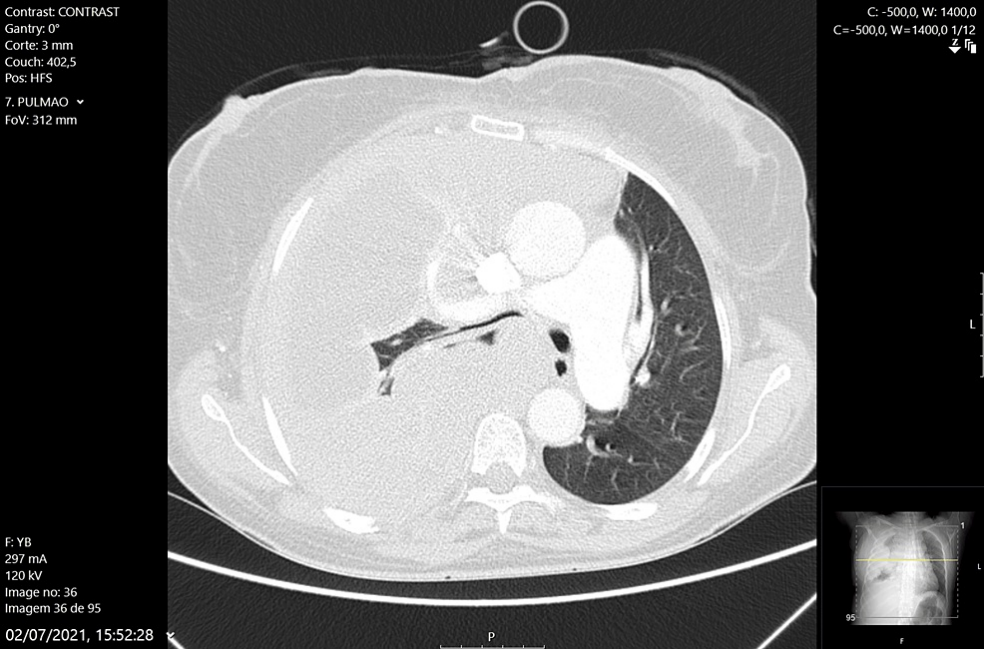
Thoracic CT scan showing almost full involvement on the right side
